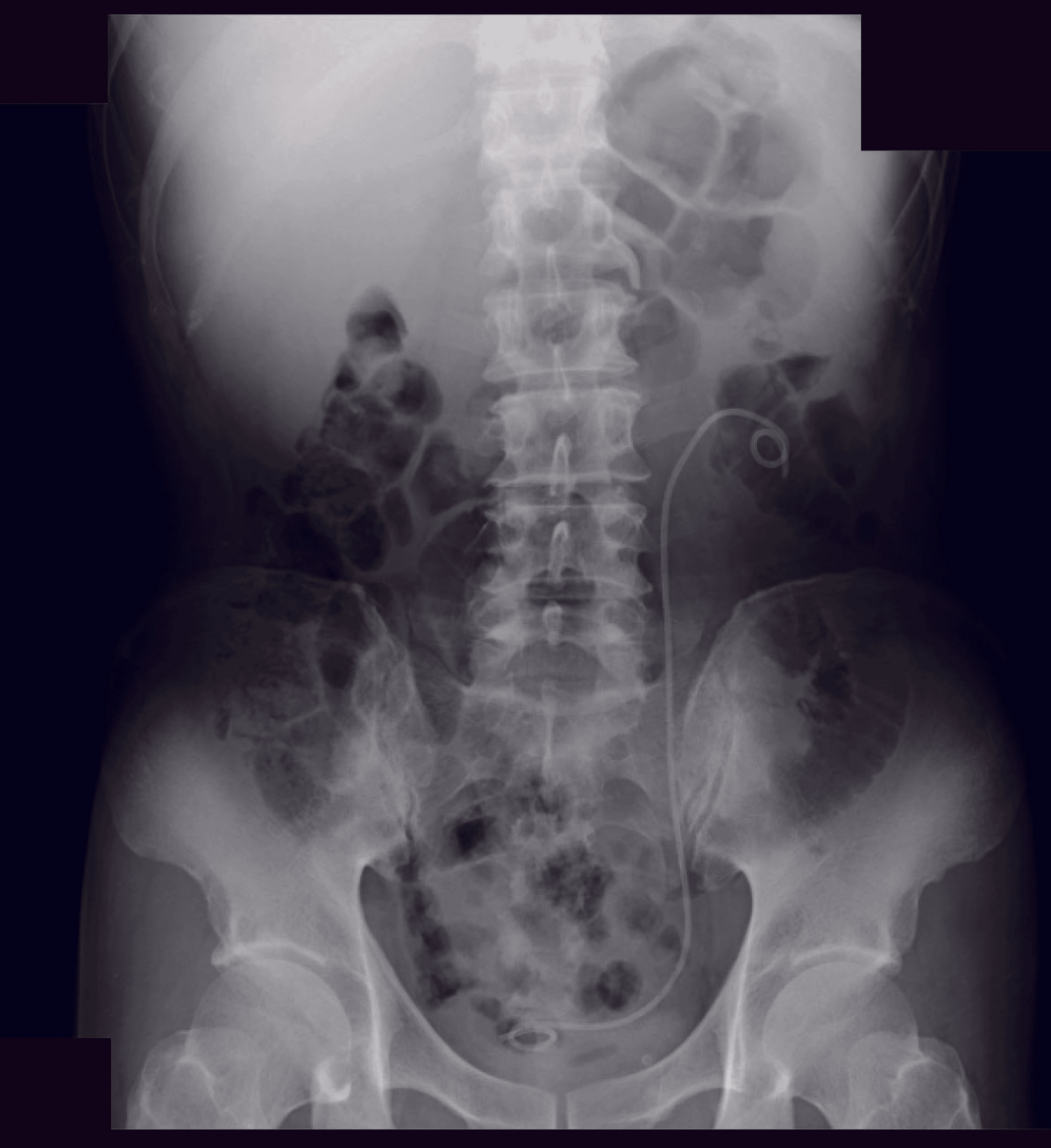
Plain abdominal radiograph obtained after placement of a double-J ureteral stent
